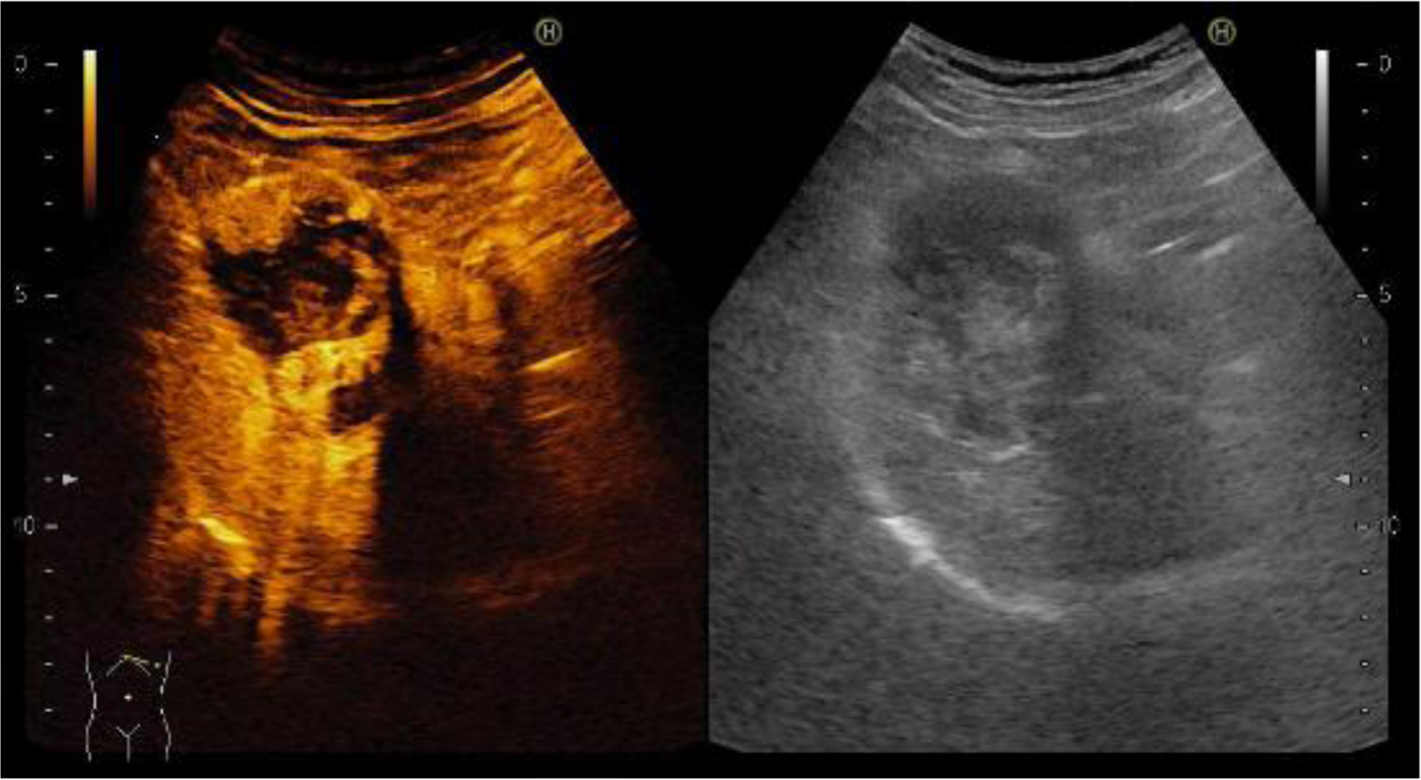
Abdominal ultrasonography findings. A 51-mm-wide tumor in hepatic segment 4 with heterogeneous echo was detected and it didn't present bloodstream increase. Sonazoid enhanced ultrasonography with hypervolemic contrasting pattern revealed that the tumor was enhanced in the early phase and washed out in the late phase
